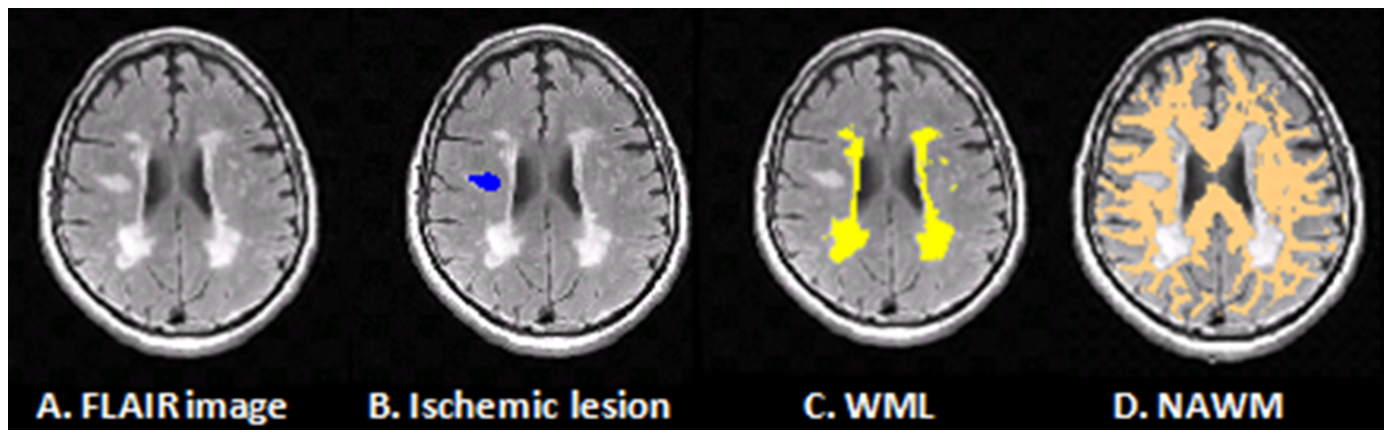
Tissue segmentation example. Subtext: A 60 year old male, scanned two days following stroke onset (A) FLAIR, fluid-attenuated inversion recovery image; (B) Ischemic lesion (blue); (C) WML, white matter lesions (yellow); (D) NAWM, normal appearing white matter (copper).
